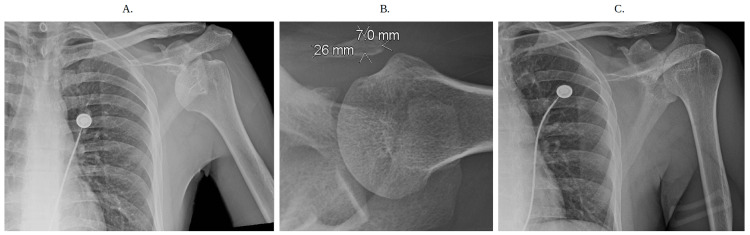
Initial X-rays of the left shoulder at the emergency department on presentation (a) There is a partial anterior and inferior dislocation of the humeral head without fracture with the acromioclavicular joint which is maintained. (b) There is a 2.6 × 0.7 cm corticated osseous density in the anterior region of the shoulder which may be due to old trauma. (c) Figure shown displays proper reduction of the dislocated left humeral head
